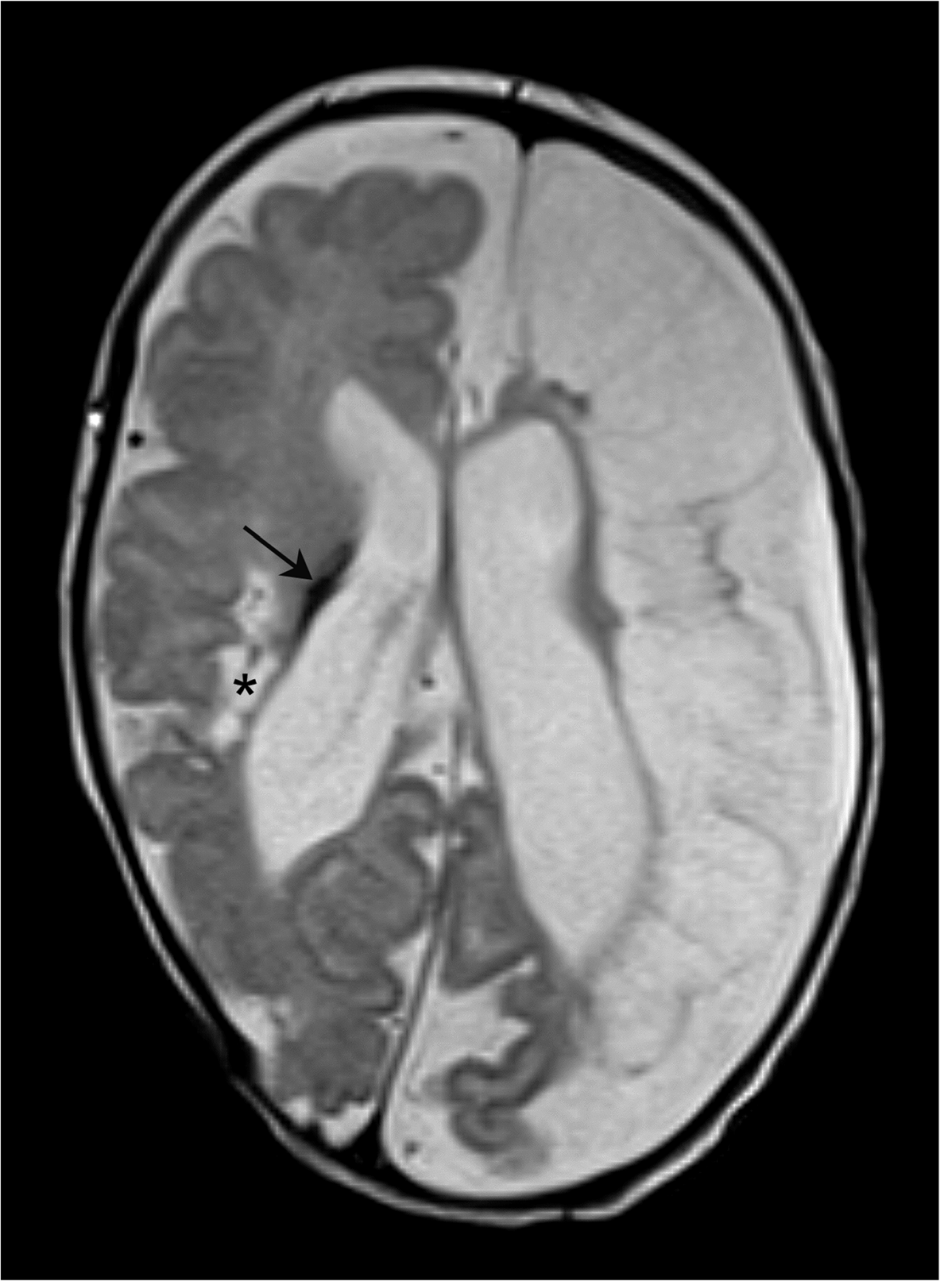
Cerebral MRI scan at term equivalent age showing a postischaemic cystic encephalomalacia of the left hemisphere and the intraventricular haemorrhage (*arrow*) with posthaemorrhagic cysts (*) on the right side with a consequent e vacuo dilatation of the lateral ventricles
